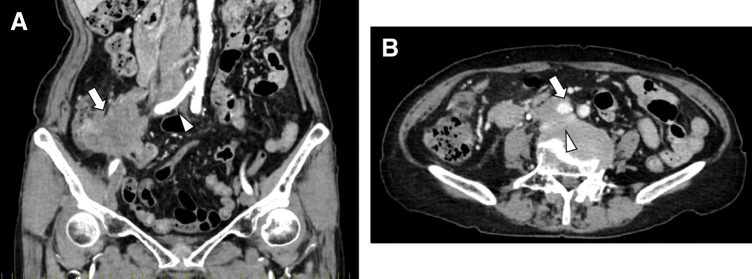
(A) Coronal contrast-enhanced CT image on initial presentation showing an exophytic tumor arising from the cecum (arrow), with enlarged para-aortic lymph nodes and invasion of the right common iliac artery (arrowhead). (B) Axial contrast-enhanced CT image on initial presentation showing a metastatic para-aortic lymph node invading the right common iliac artery (arrow) and extending anteriorly to involve the inferior vena cava (arrowhead).
